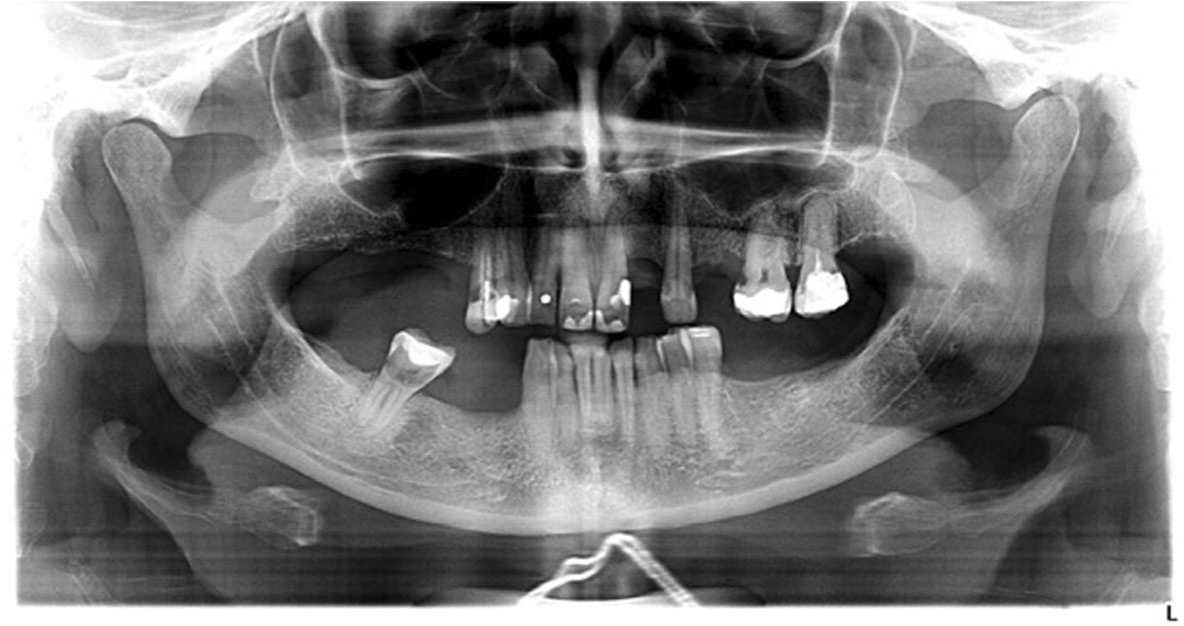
Panoramic radiograph with C1 category of MCI
